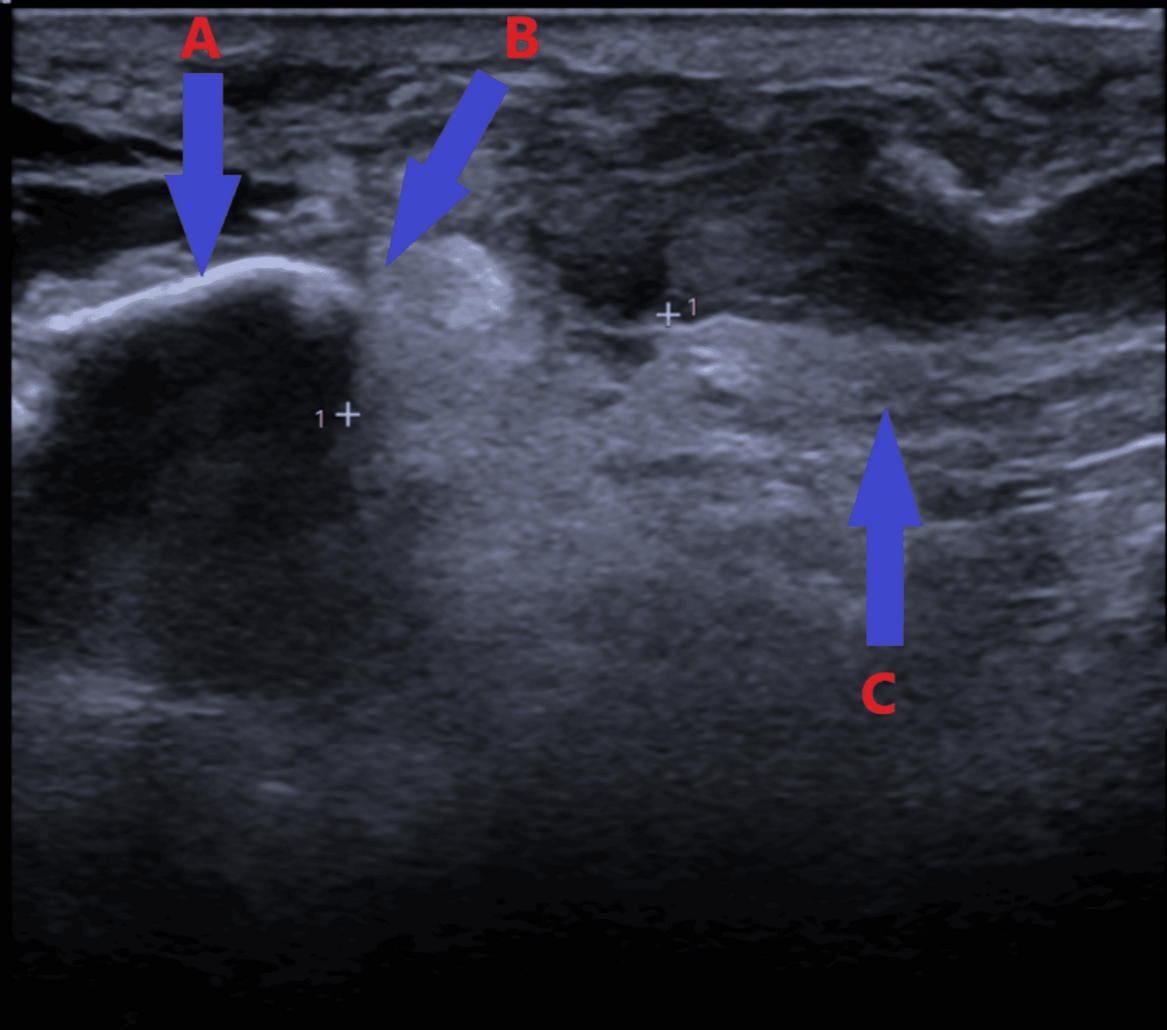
Ultrasound scan image showing avulsion of the patella tendon from the inferior pole of the patella: (A) patella, (B) avulsion fracture of the distal pole of the patella, and (C) patella tendon
